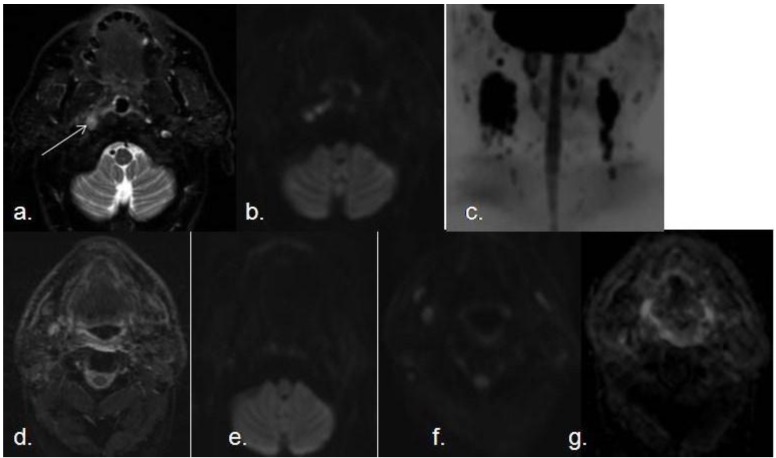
Pre- and post-reatment SCC with lymph node metastasis. (a) Transverse STIR shows a small nodular hyperintense lesion in right torus tubarius; (b) The lesion demonstrates increased signal intensity on DWI with high b value (white arrow); (c) Coronal MIP of a DWIBS sequence with high b value demonstrates several bilateral enlarged lymph nodes with restricted diffusion The lesion was biopsied and confirmed as a SCC with bilateral metastatic lymph nodes. Postreatment follow-up MRI (six months after radio and chemotherapy); (d) axial STIR; (e and f) axial DWI with high b value at two different levels; (g) corresponding ADC map, demonstrates absence of recurrence, with no areas of restricted diffusion or suspicious lymph nodes.
